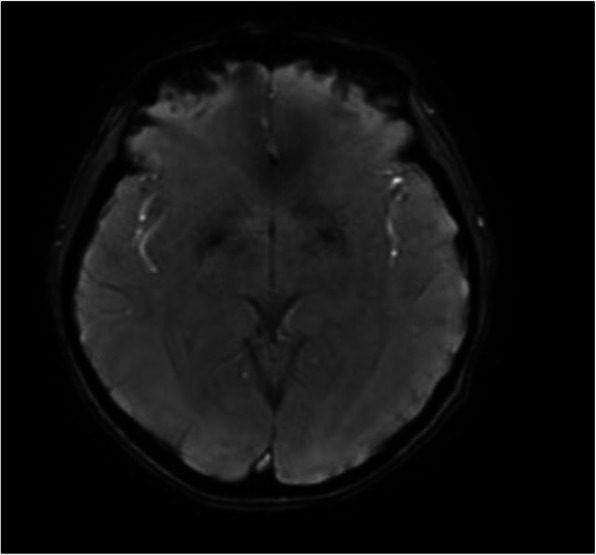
Proband (III-1) MRI brain susceptibility weighted imaging demonstrates moderate symmetric hypointensity in the bilateral globi pallidi
